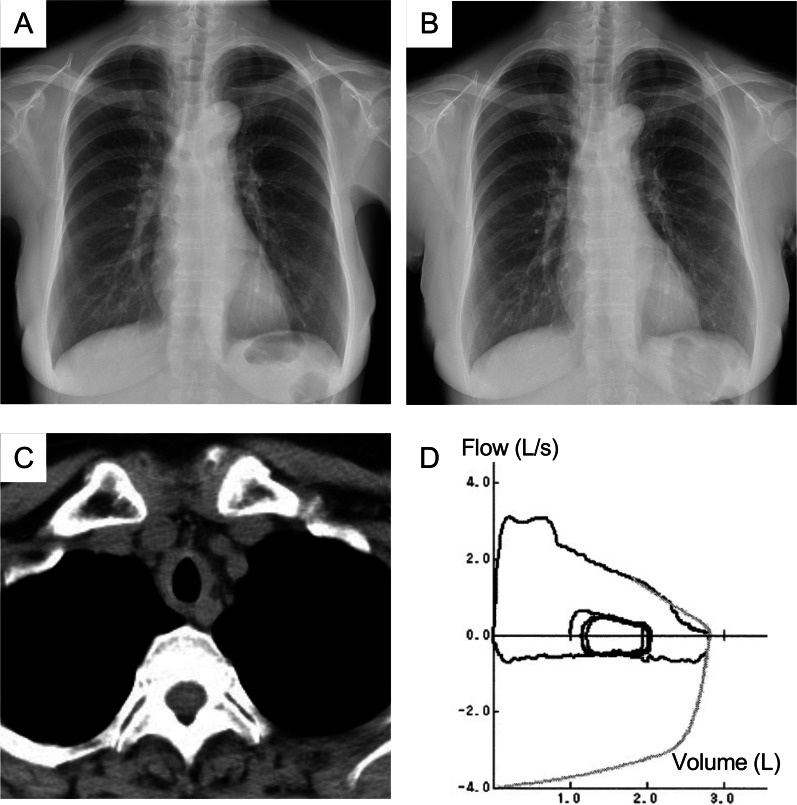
Chest X-rays obtained at the first visit (A) and 1 year before the first visit (B), respectively. Chest X-ray at the first visit (A) showing narrowing of the trachea compared with that from a year earlier (B). C Chest computed tomography image showing circumferential thickening of the tracheal wall. D Flow–volume loop graph demonstrating fixed upper airway obstruction
